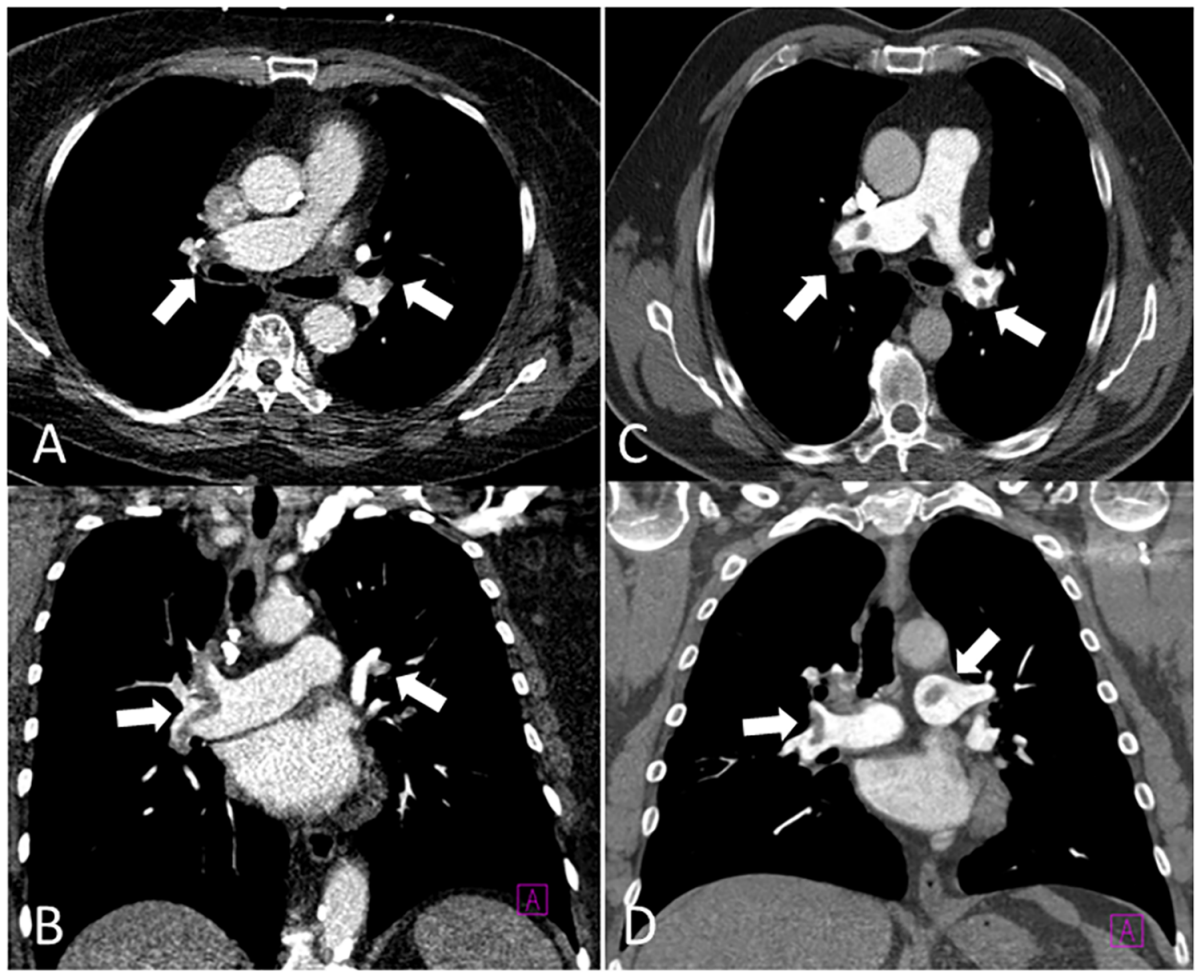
Axial and coronal CT images of the pulmonary arteries. On the left side (A + B) there is a CTPA acquired with 80 kVp and 25 ml contrast agent in a 55-year-old man with central and peripheral pulmonary emboli (see arrows). Additionally to the pulmonary arteries there is a sufficient opacification of the aorta. The CTPA on the right side (C + D) was acquired with 100 kVp and 60 ml contrast agent in a 55-year-old man with central and peripheral pulmonary emboli (see arrows). While the standard protocol shows a better image quality in terms of less image noise, the low dose and low contrast media protocol is feasible to detect a pulmonary embolism and pathologies in the aorta.
